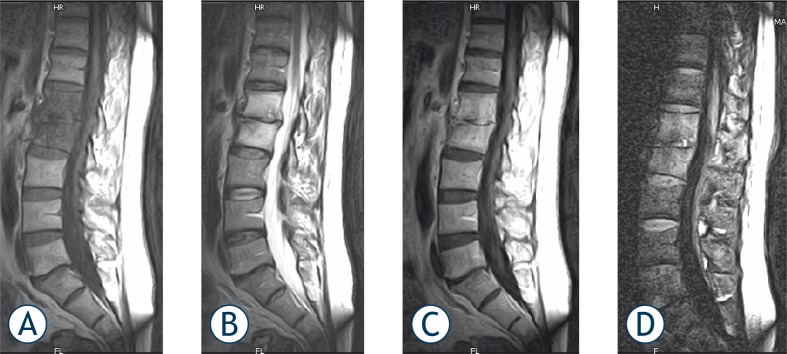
Sagittal MR images from 38 years old male patient with acute spondylodiscitis. A) T1-weighted image showing complete homogenous hypointensity at the L1 and L2 vertebrae corpus and adjacent intervertebral disc. There are also osteophytic changes on end-plate and loss of L1–2 intervertebral disc space. B) T2-weighted images showing hyperintense signal intensity corresponding to the same level. C) Postcontrast image showing homogenous enhancement of disc and adjacent vertebrae corpus. D) DWI revealing high signal intensity relative to neighbouring normal vertebrae.
